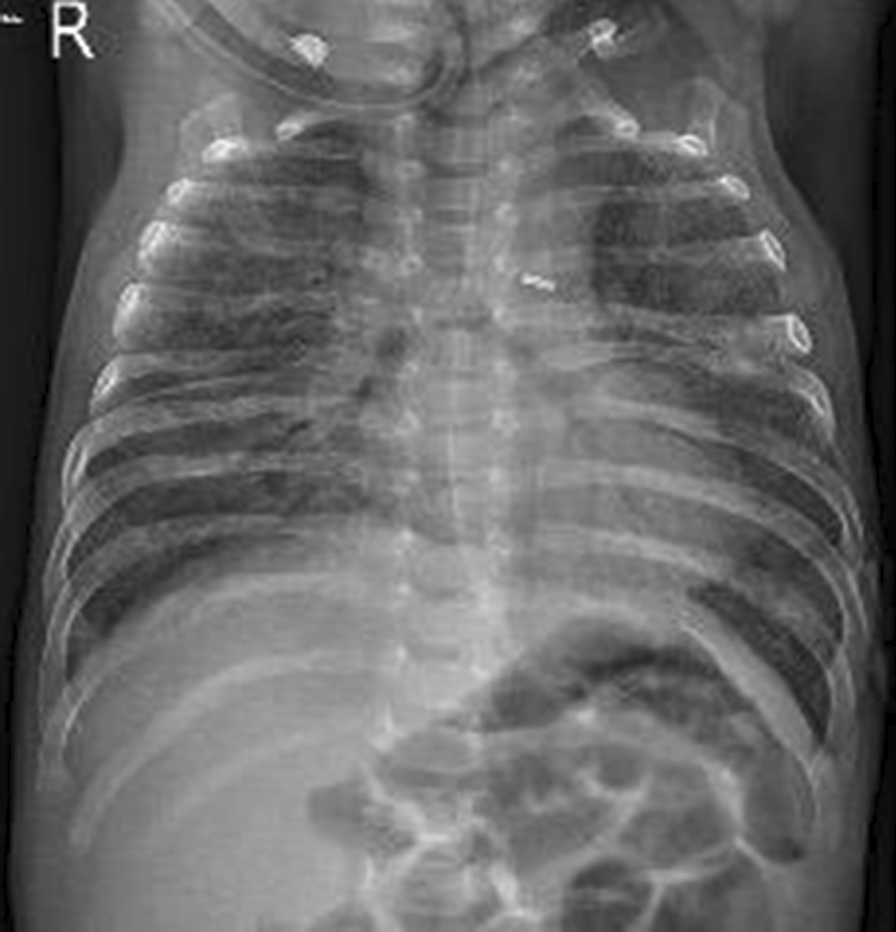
Patient’s chest X-ray after receiving hydroxychloroquine and just before discharge
